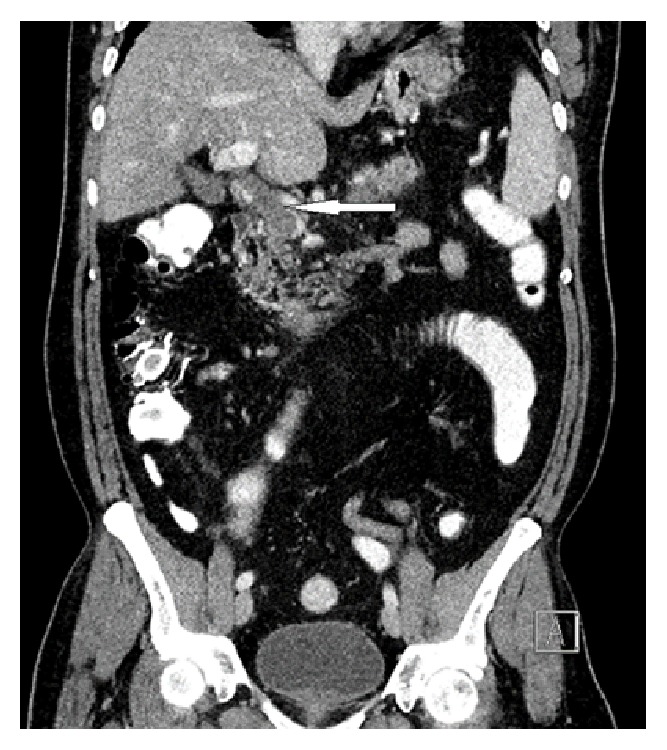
Abdomen CT scan with contrast (reformatted coronal image) shows distended portal confluence with filling defect occluding its lumen (white arrow).
